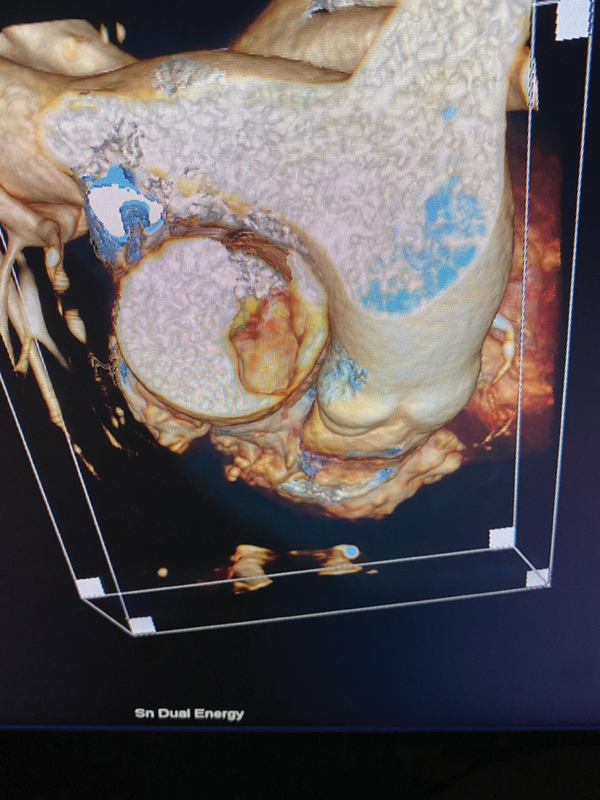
Computed tomography angiography mass in ascending aorta.
